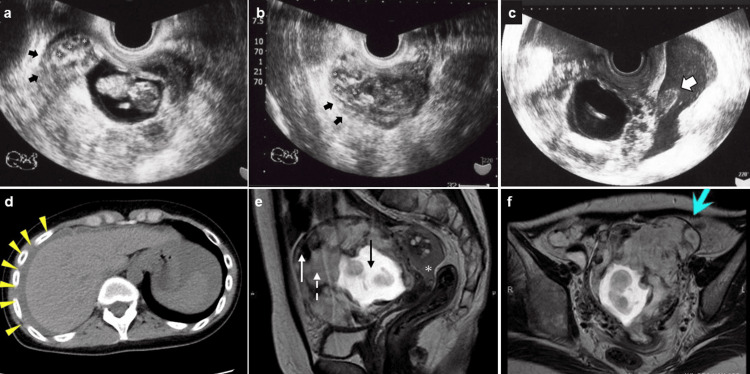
Sagittal sonographic image of the fundal placenta at 10 weeks of gestation Note the placental lacunae marked with asterisks (*) producing a “moth-eaten” appearance of the placenta (a) and the absence of the normal retroplacental sonolucency, or clearly detectable myometrium (black arrows) (b). Placental bulge protruding from the uterus is confirmed as bloody ascites (c). Bloody ascites around the liver, derived from unknown origin, are observed on abdominal computed tomography (yellow triangles) (d). The sagittal T2-weighted image shows the fetus (black arrow) and persistent high signal intensity of the placenta (dashed white arrow) with the low signal intensity of the adjacent myometrium (solid white arrow) with bloody ascites in the Douglas pouch (white asterisk) (e). Axial T2-weighted image shows a partial bulging of the placenta outside the uterus, a sign of “placental bulging” (blue arrow) (f).
